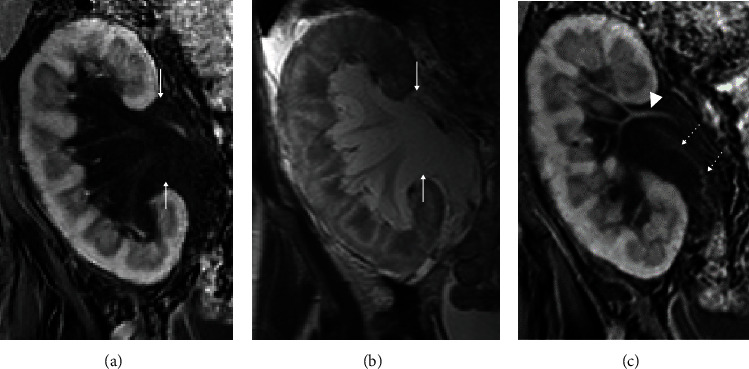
Coronal MRI of transplanted kidney. Postcontrast fat-saturated (a, c) and T2-weighted (b) show a nonenhancing peripelvic multiseptated fluid collection centered in the renal hilum which is hypointense on T1-weighted images and hyperintense on T2-weighted images (arrows, (a, b)). The fluid collection is encasing the hilar vasculature (arrowhead, (c)) and insinuating parallel to the ureter (dotted arrow, (c)) but is separate from the collecting system. There is no vascular displacement or hydronephrosis.
